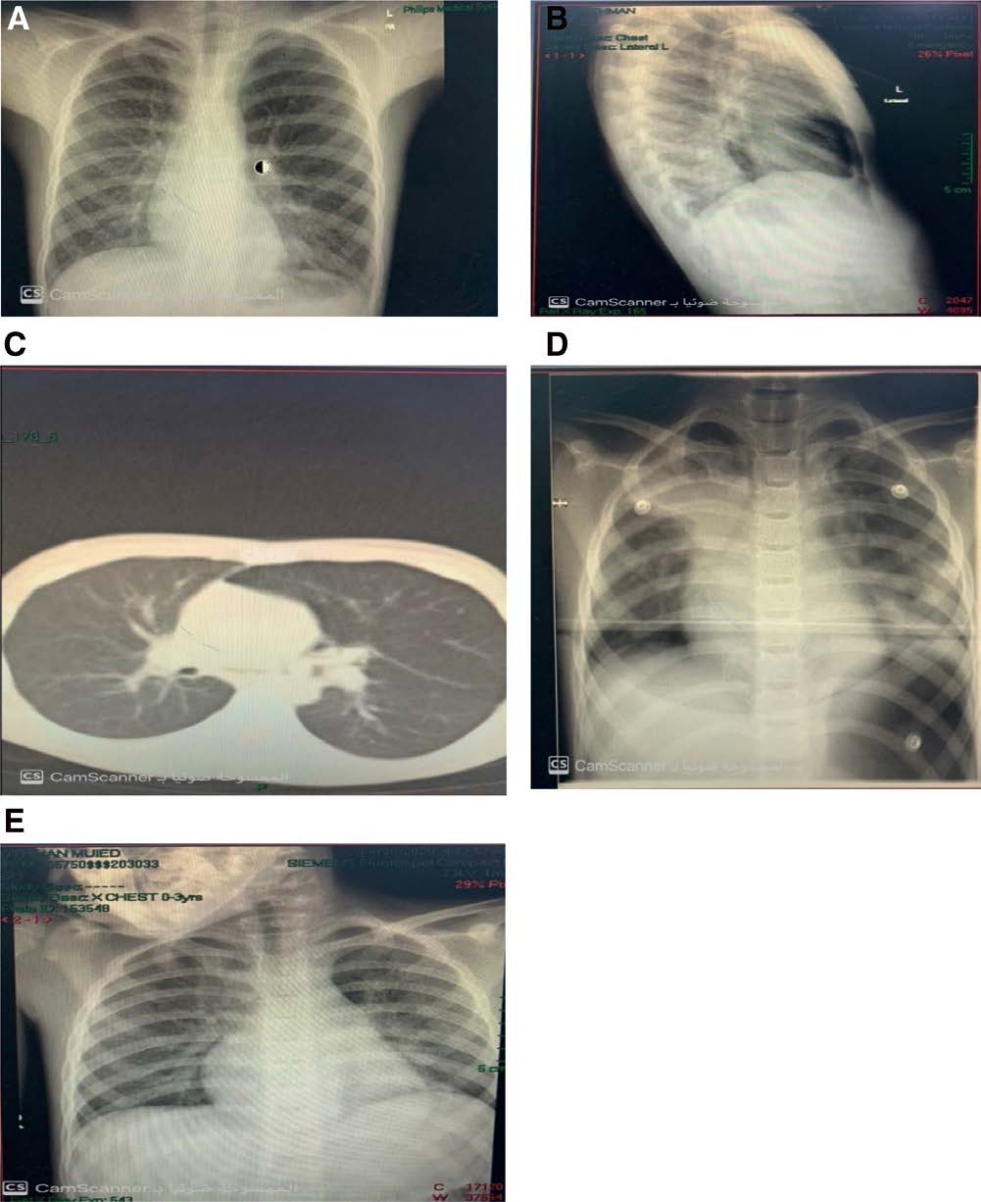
Chest radiography and CT scan were performed for case 1 before, during, and after FB removal. (A) Preoperative chest radiograph AP view. (B) Preoperative lateral chest radiograph view. (C) Axial CT chest with pneumomediastinum. (D) Intraoperative chest radiograph. (E) Postoperative 6 hours chest radiograph. CT = computed tomography.
